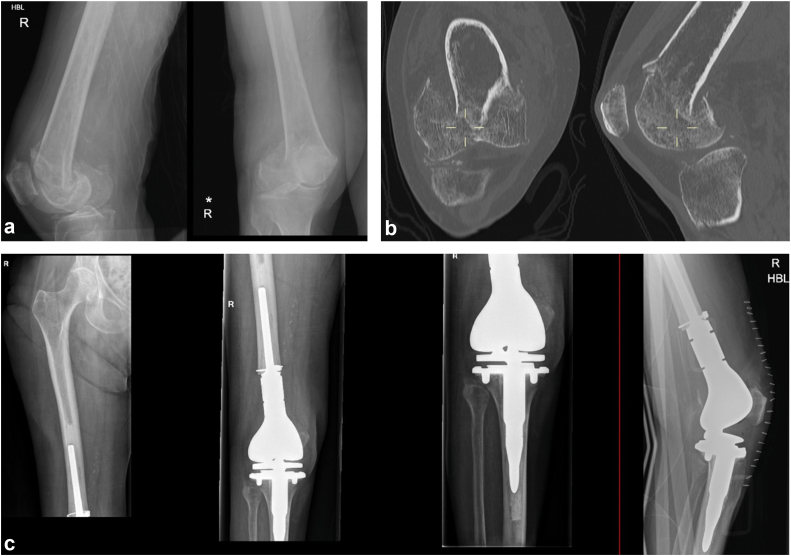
Anteroposterior and lateral radiographs of an AO 33C3 native distal femoral fracture in a 92-year-old female, managed with distal femoral endoprosthetic replacement. (a) Anteroposterior and lateral radiographs of distal femoral fracture. (b) Relevant coronal and sagittal computed tomography images of the same distal femoral fracture. (c) Anteroposterior and lateral postoperative radiographs following distal femoral endoprosthetic replacement in the same patient.
